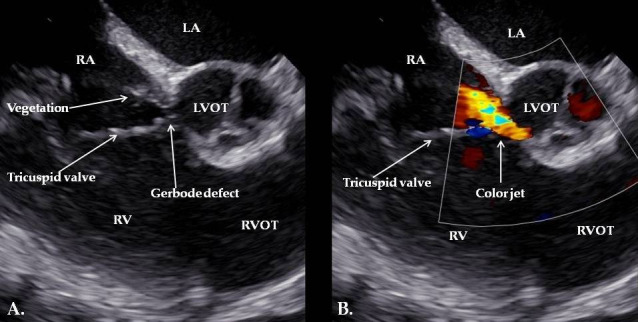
Two-dimensional transesophageal echocardiogram shows a Gerbode defect, along with a mobile, echogenic and filiform mass attached near the tricuspid valve (measuring 19 × 6 mm) (A). Color Doppler imaging demonstrates an abnormal, high-velocity systolic jet extending from the left ventricle into the right atrium. This finding confirms the presence of a left ventricle-to-right atrium communication, characteristic of a Gerbode defect (B). LA: Left Atrium; RA: Right Atrium; RV: Right Ventricle; LVOT: Left Ventricular Outflow Tract; RVOT: Right Ventricular Outflow Tract.
